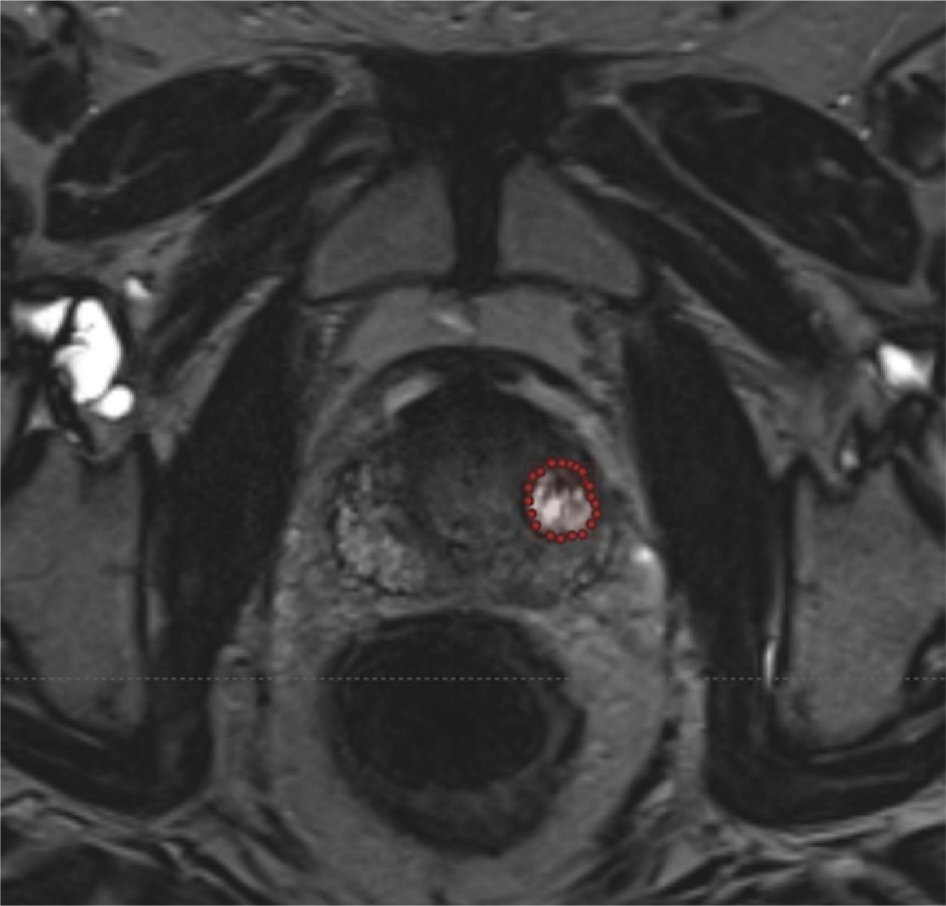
Axial t2-weighted MR image showing subtle low-signal-intensity area in anterior peripheral zone. Source: [28]
